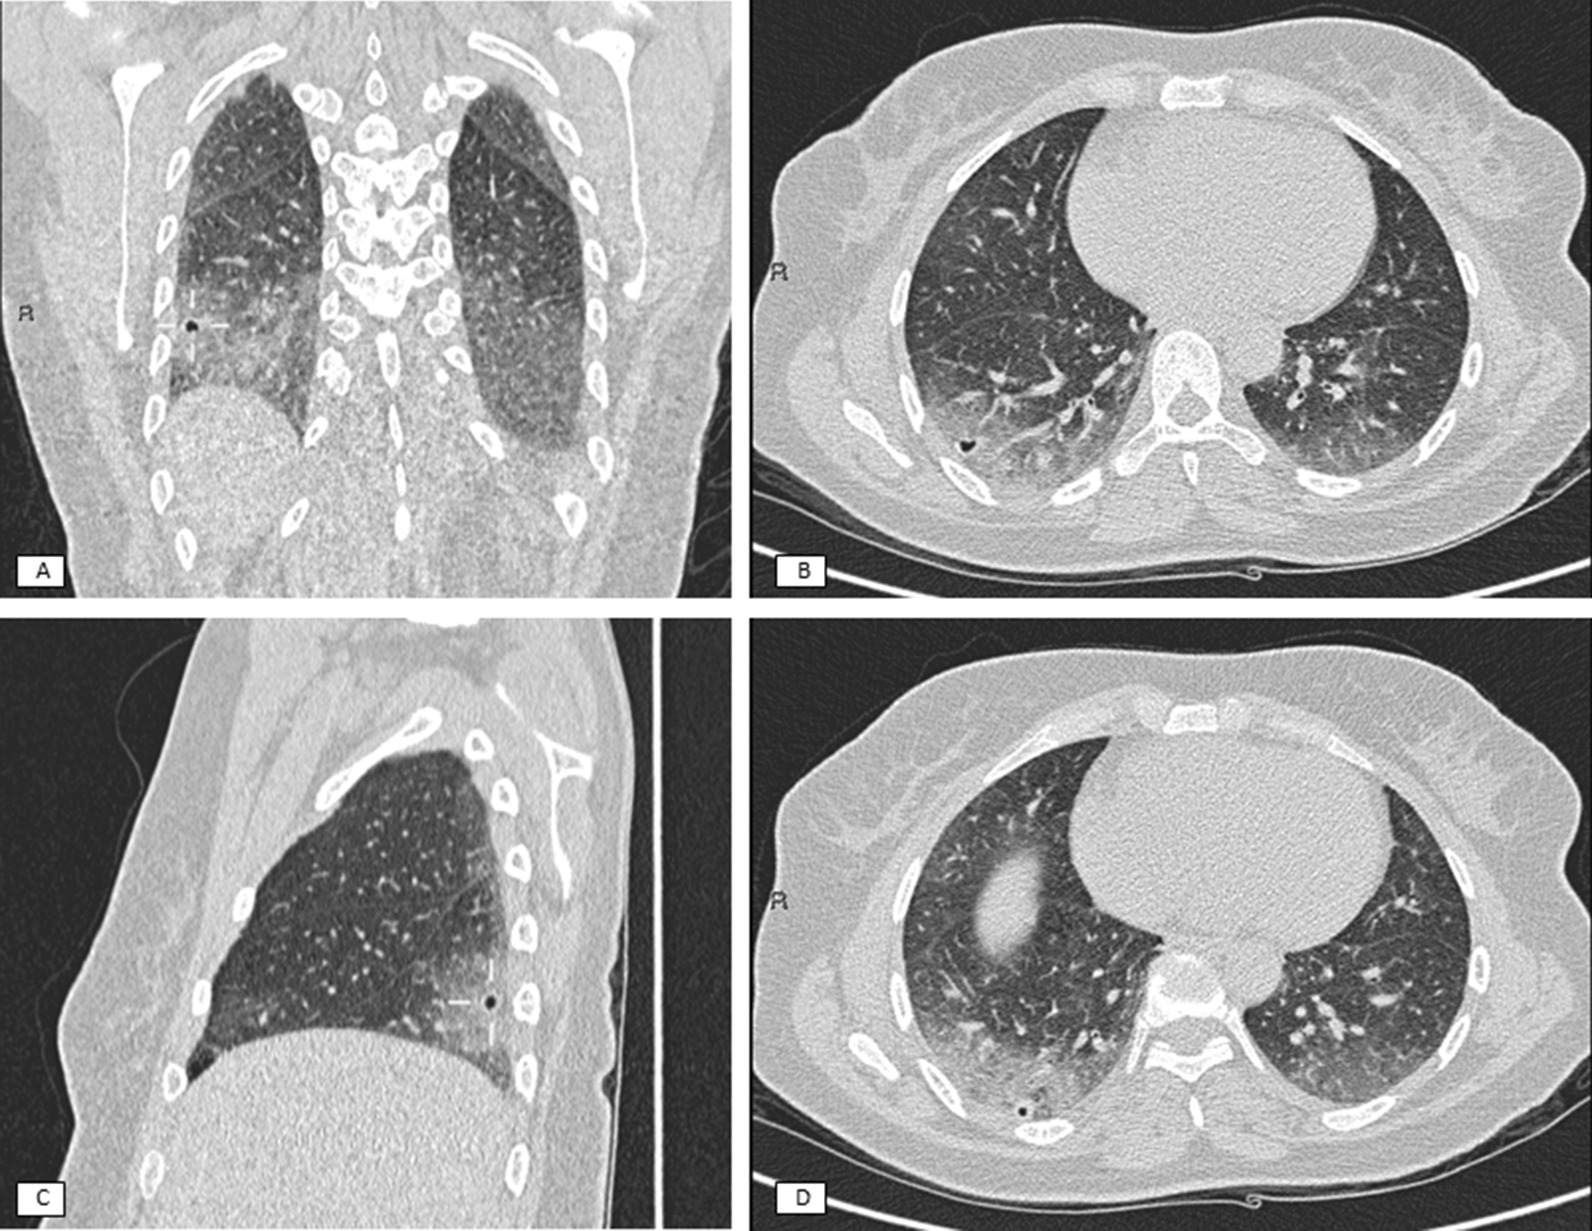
CT scan five days after cryobiopsies. a Lateral pneumatocele (arrows) in coronal plane. b Lateral pneumatocele in sagittal plane. c Lateral pneumatocele (arrows) in axial plane. d Medial pneumatocele in axial plane
